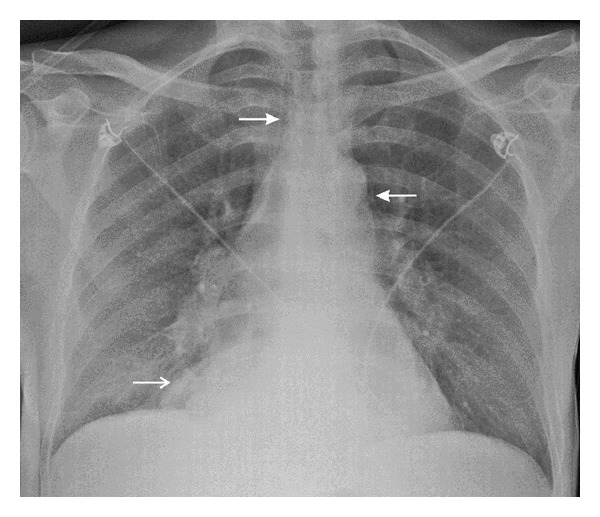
Chest X-ray at admission at the emergency ward showing a pneumomediastinum (closed arrows) and silhouette sign over the right heart border (open arrow). No pleural effusions were observed in the costolateral sinuses.
